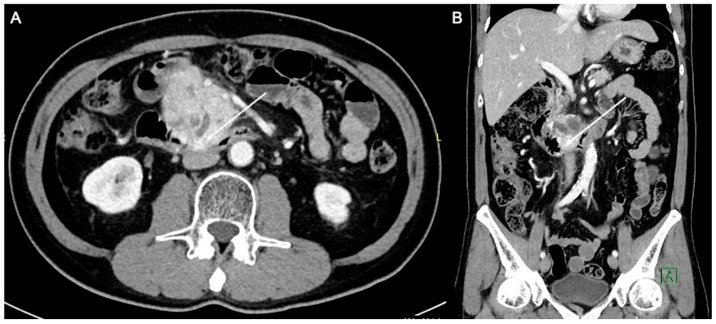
Axial (A) and coronal (B) computed tomography images of the patient demonstrating a heterogeneously enhancing pancreatic mass measuring approximately 6.3 × 4.4 cm. There is duodenal involvement by the pancreatic mass (arrows).
